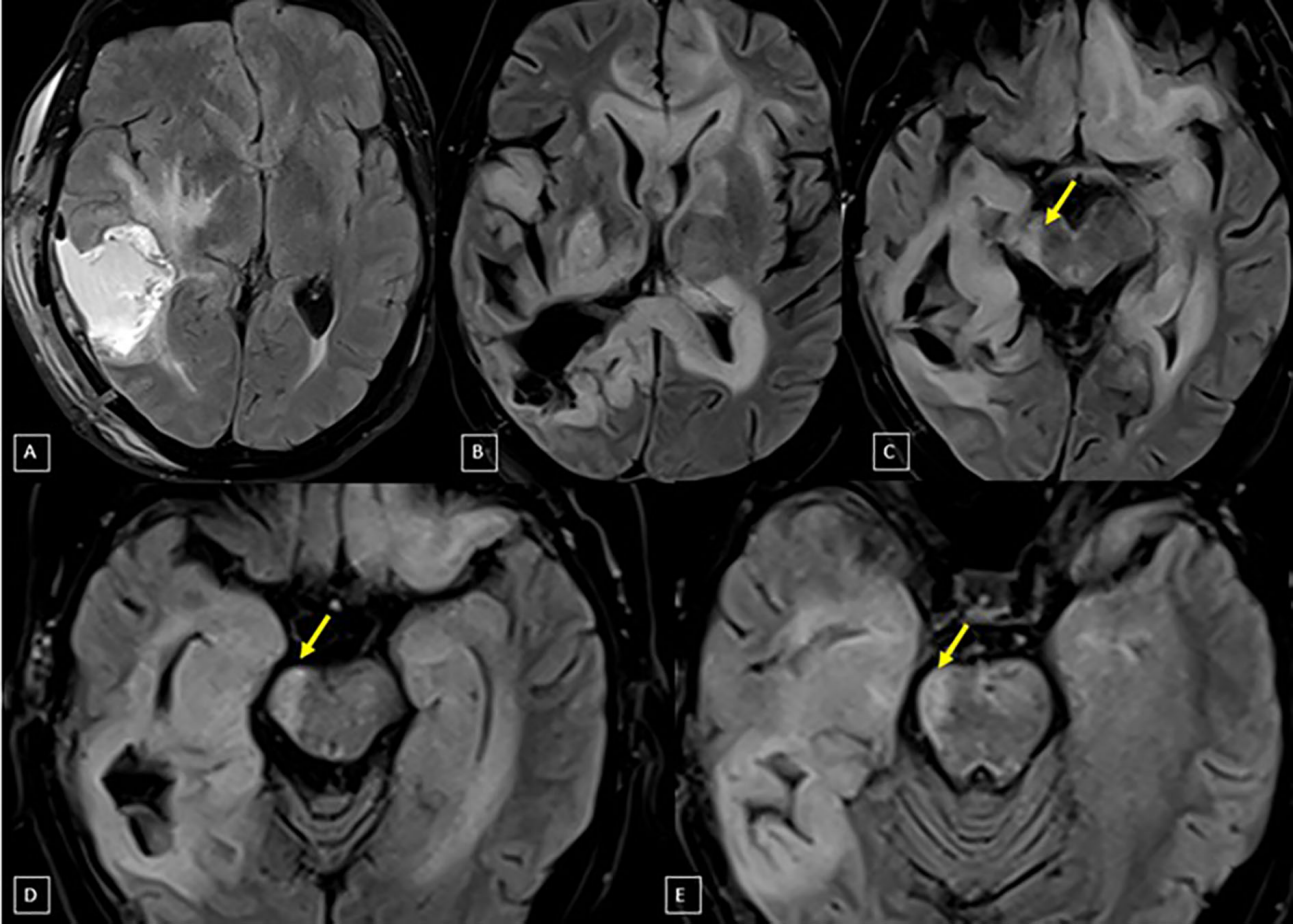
Axial plane MRI studies with FLAIR sequence: The first image (A) was taken post-surgery and before radiotherapy, showing the right temporoparietal surgical cavity and an adjacent area of signal alteration representing edema/tumor infiltration. The following images (B–E – superior to inferior) are from the follow-up, five years later, demonstrating volumetric reduction (arrows) affecting the right cerebral peduncle and midbrain tegmentum, extending to the right medullary pyramid, with an atrophic appearance likely related to treatment – suggestive of WDCT.
